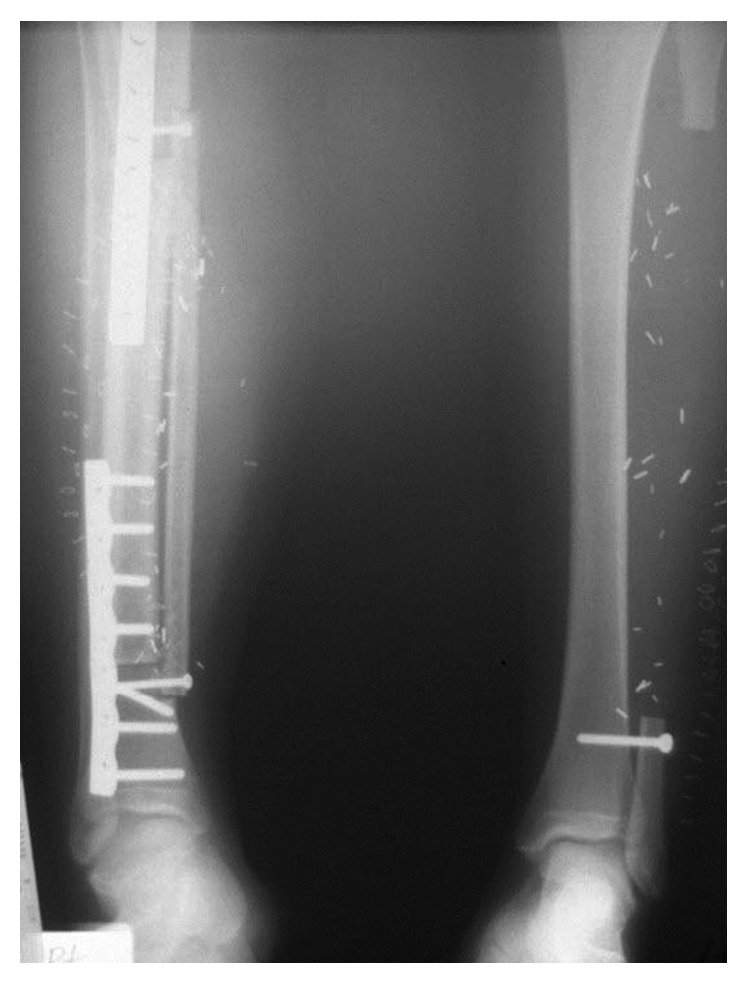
An anteroposterior radiograph of the bilateral lower legs following adamantinoma resection and limb reconstruction. On the right, the allograft is positioned laterally with the vascularized fibular graft placed overlying it medially. The grafts are fixed by plates and screws. On the left, the fibula has been resected and the ankle mortise has been stabilized proximally with a screw.
